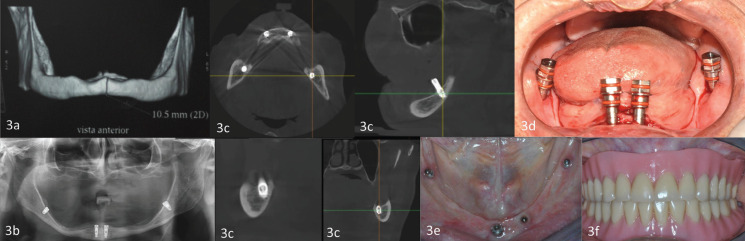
a) Tomographic image. Clinical case. b) Panoramic radiograph after implant placement. c) Tomographic image with implants in place. Clinical case. d) Clinical image of patient with implants. e) Image of implants in mouth. Clinical case. f) Image of patient with prosthesis. Clinical case.
